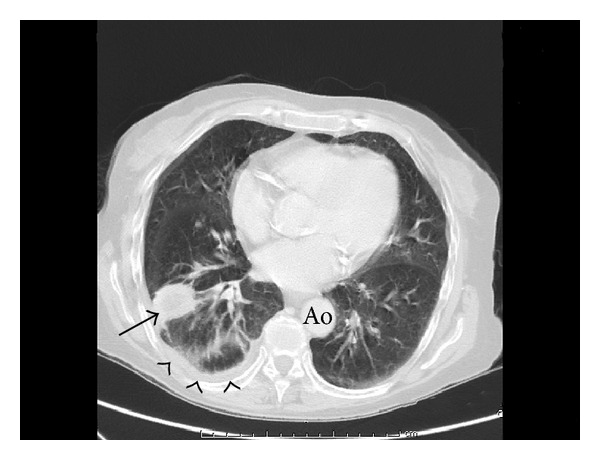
Computed tomography of the chest (lung window) showing a small focal consolidation within the posterior segment of the right lower lobe (arrow) and mild pleural effusion (arrowheads).
